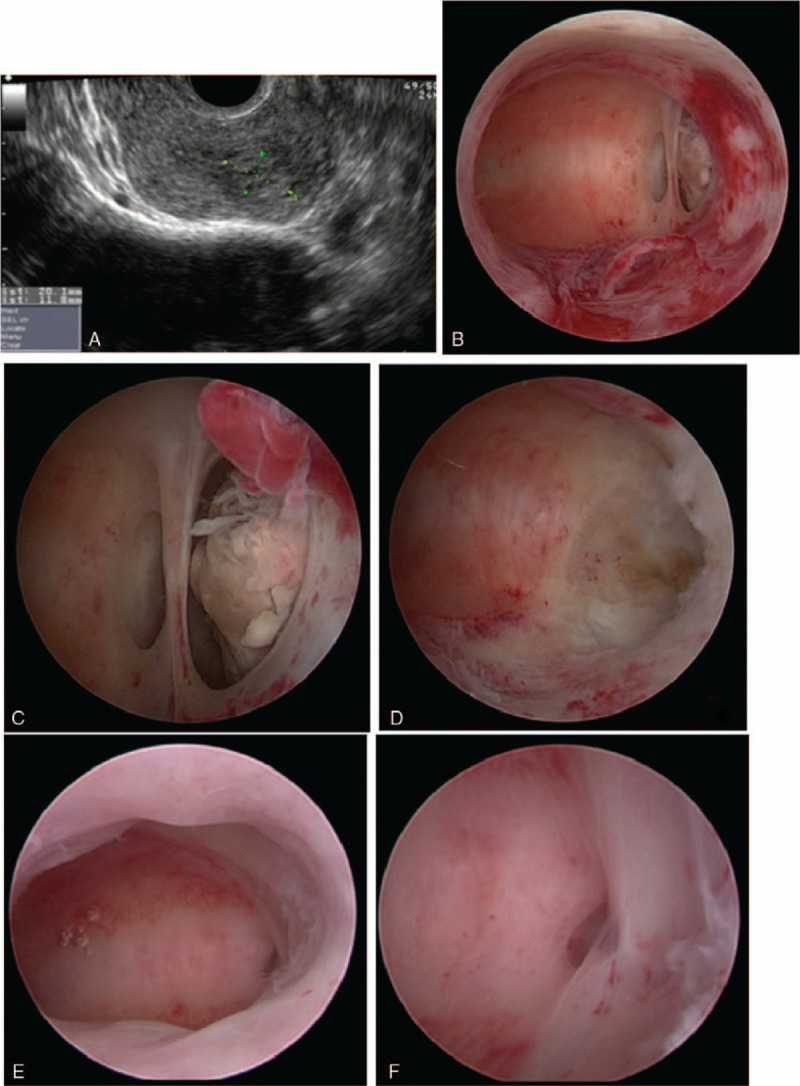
(A) ultrasound image of placental remnants, (B and C) hysteroscopic view of placental remnants in the left uterine horn, (D) hysteroscopic view of left uterine horn after complete resection of placental remnants, (E) second-look hysteroscopic view of uterine cavity, and (F) second-look hysteroscopic view of left uterine horn.
